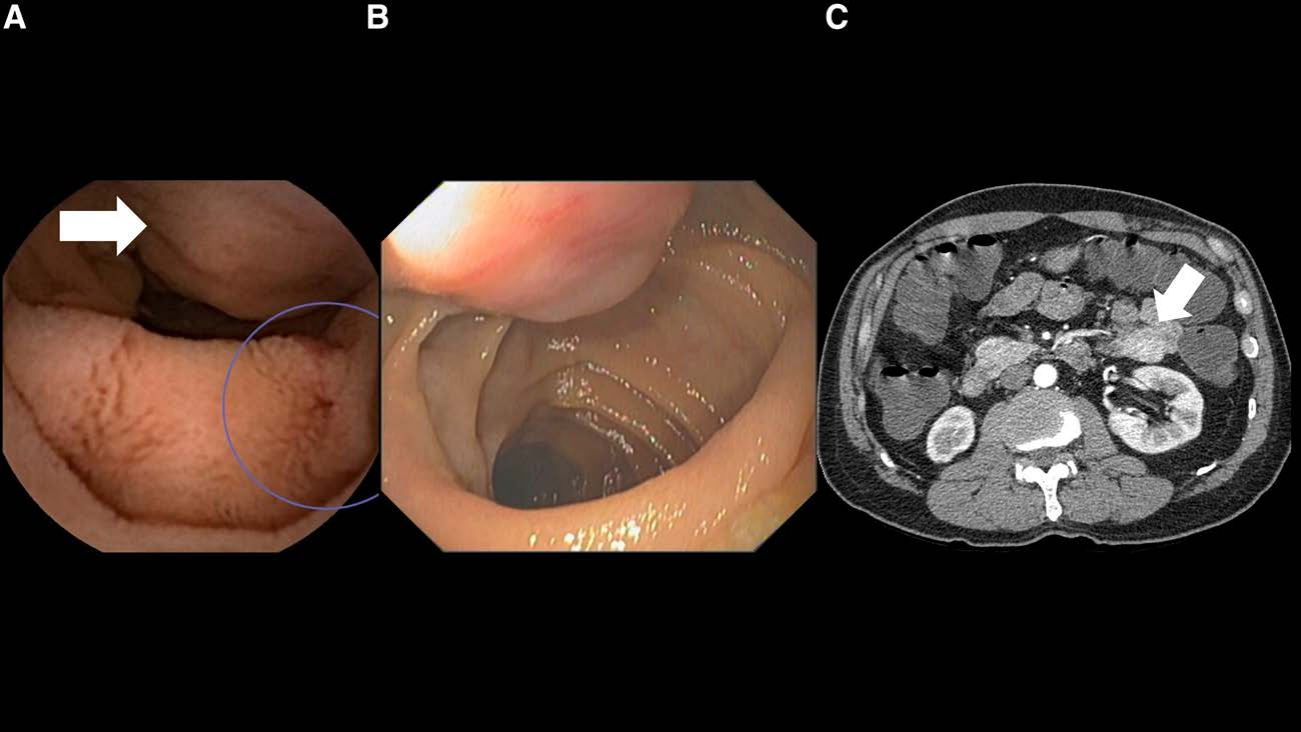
In Figure 3A, video capsule endoscopy (VCE) incorrectly reported an angiodysplasia at jejunum as a source of bleeding (purplish circle) but missed a mass beside (white arrow). The mass was discovered by balloon-assisted enteroscopy as shown in Figure 3B. CT abdomen was performed for preoperative assessment and showed a well circumscribed submucosal mass arising from collapsed proximal jejunum. The mass showed homogeneous hypervascular enhancement in Figure 3C. Surgery was performed and pathologically confirmed a gastrointestinal stromal tumor. CTE = computed tomography enterography, VCE = video capsule endoscopy.
